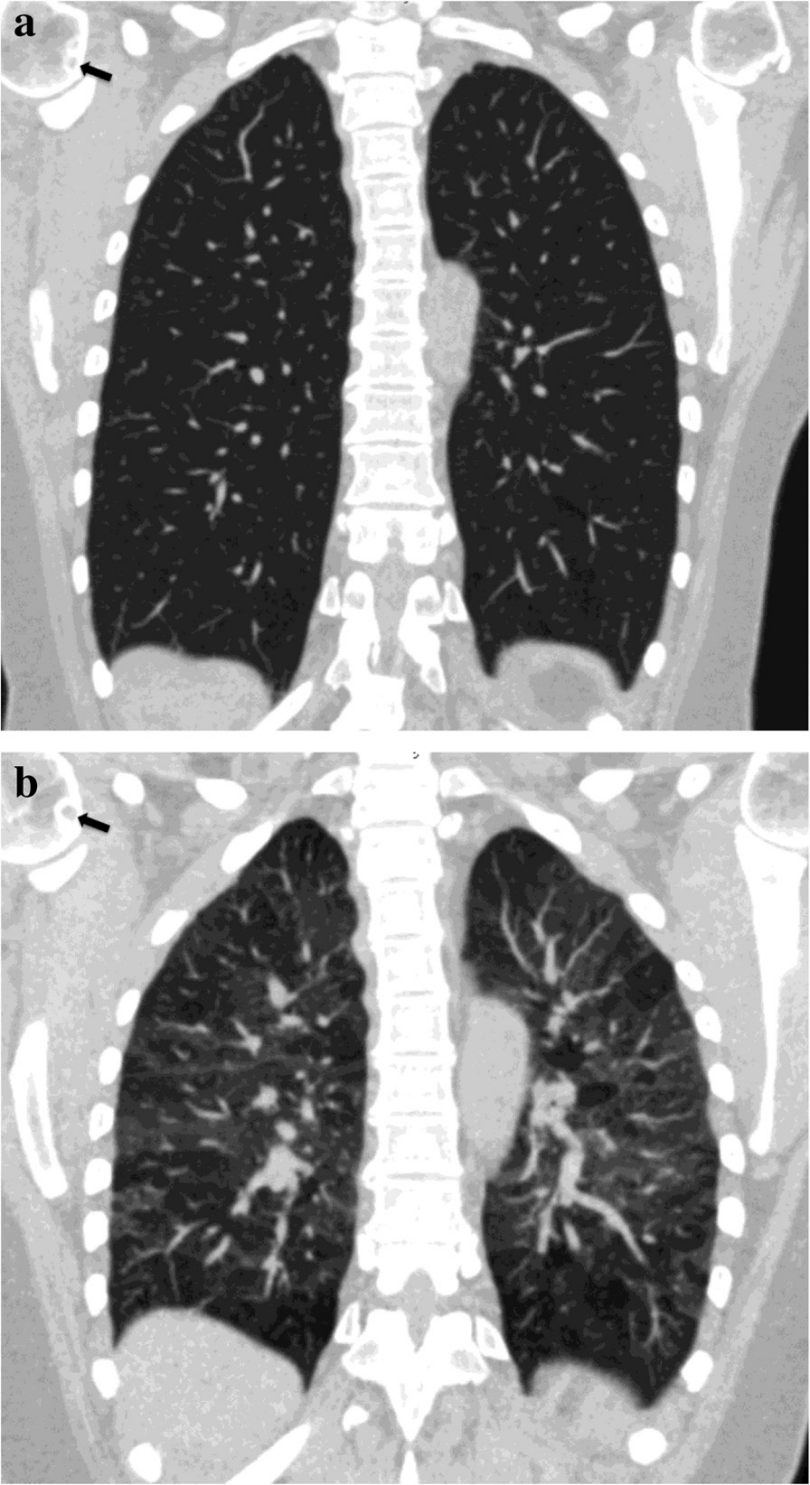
55-year-old woman with known rheumatoid arthritis complaining of exertional dyspnea, chronic cough and obstructive pattern at respiratory tests. On coronally-reformatted inspiratory CT image no lung abnormalities can be seen (a). Coronally-reformatted expiratory CT image shows extensive air-trapping (b). Note presence of subchondral cysts (arrows) and narrowing of gleno-humeral joint spaces.
